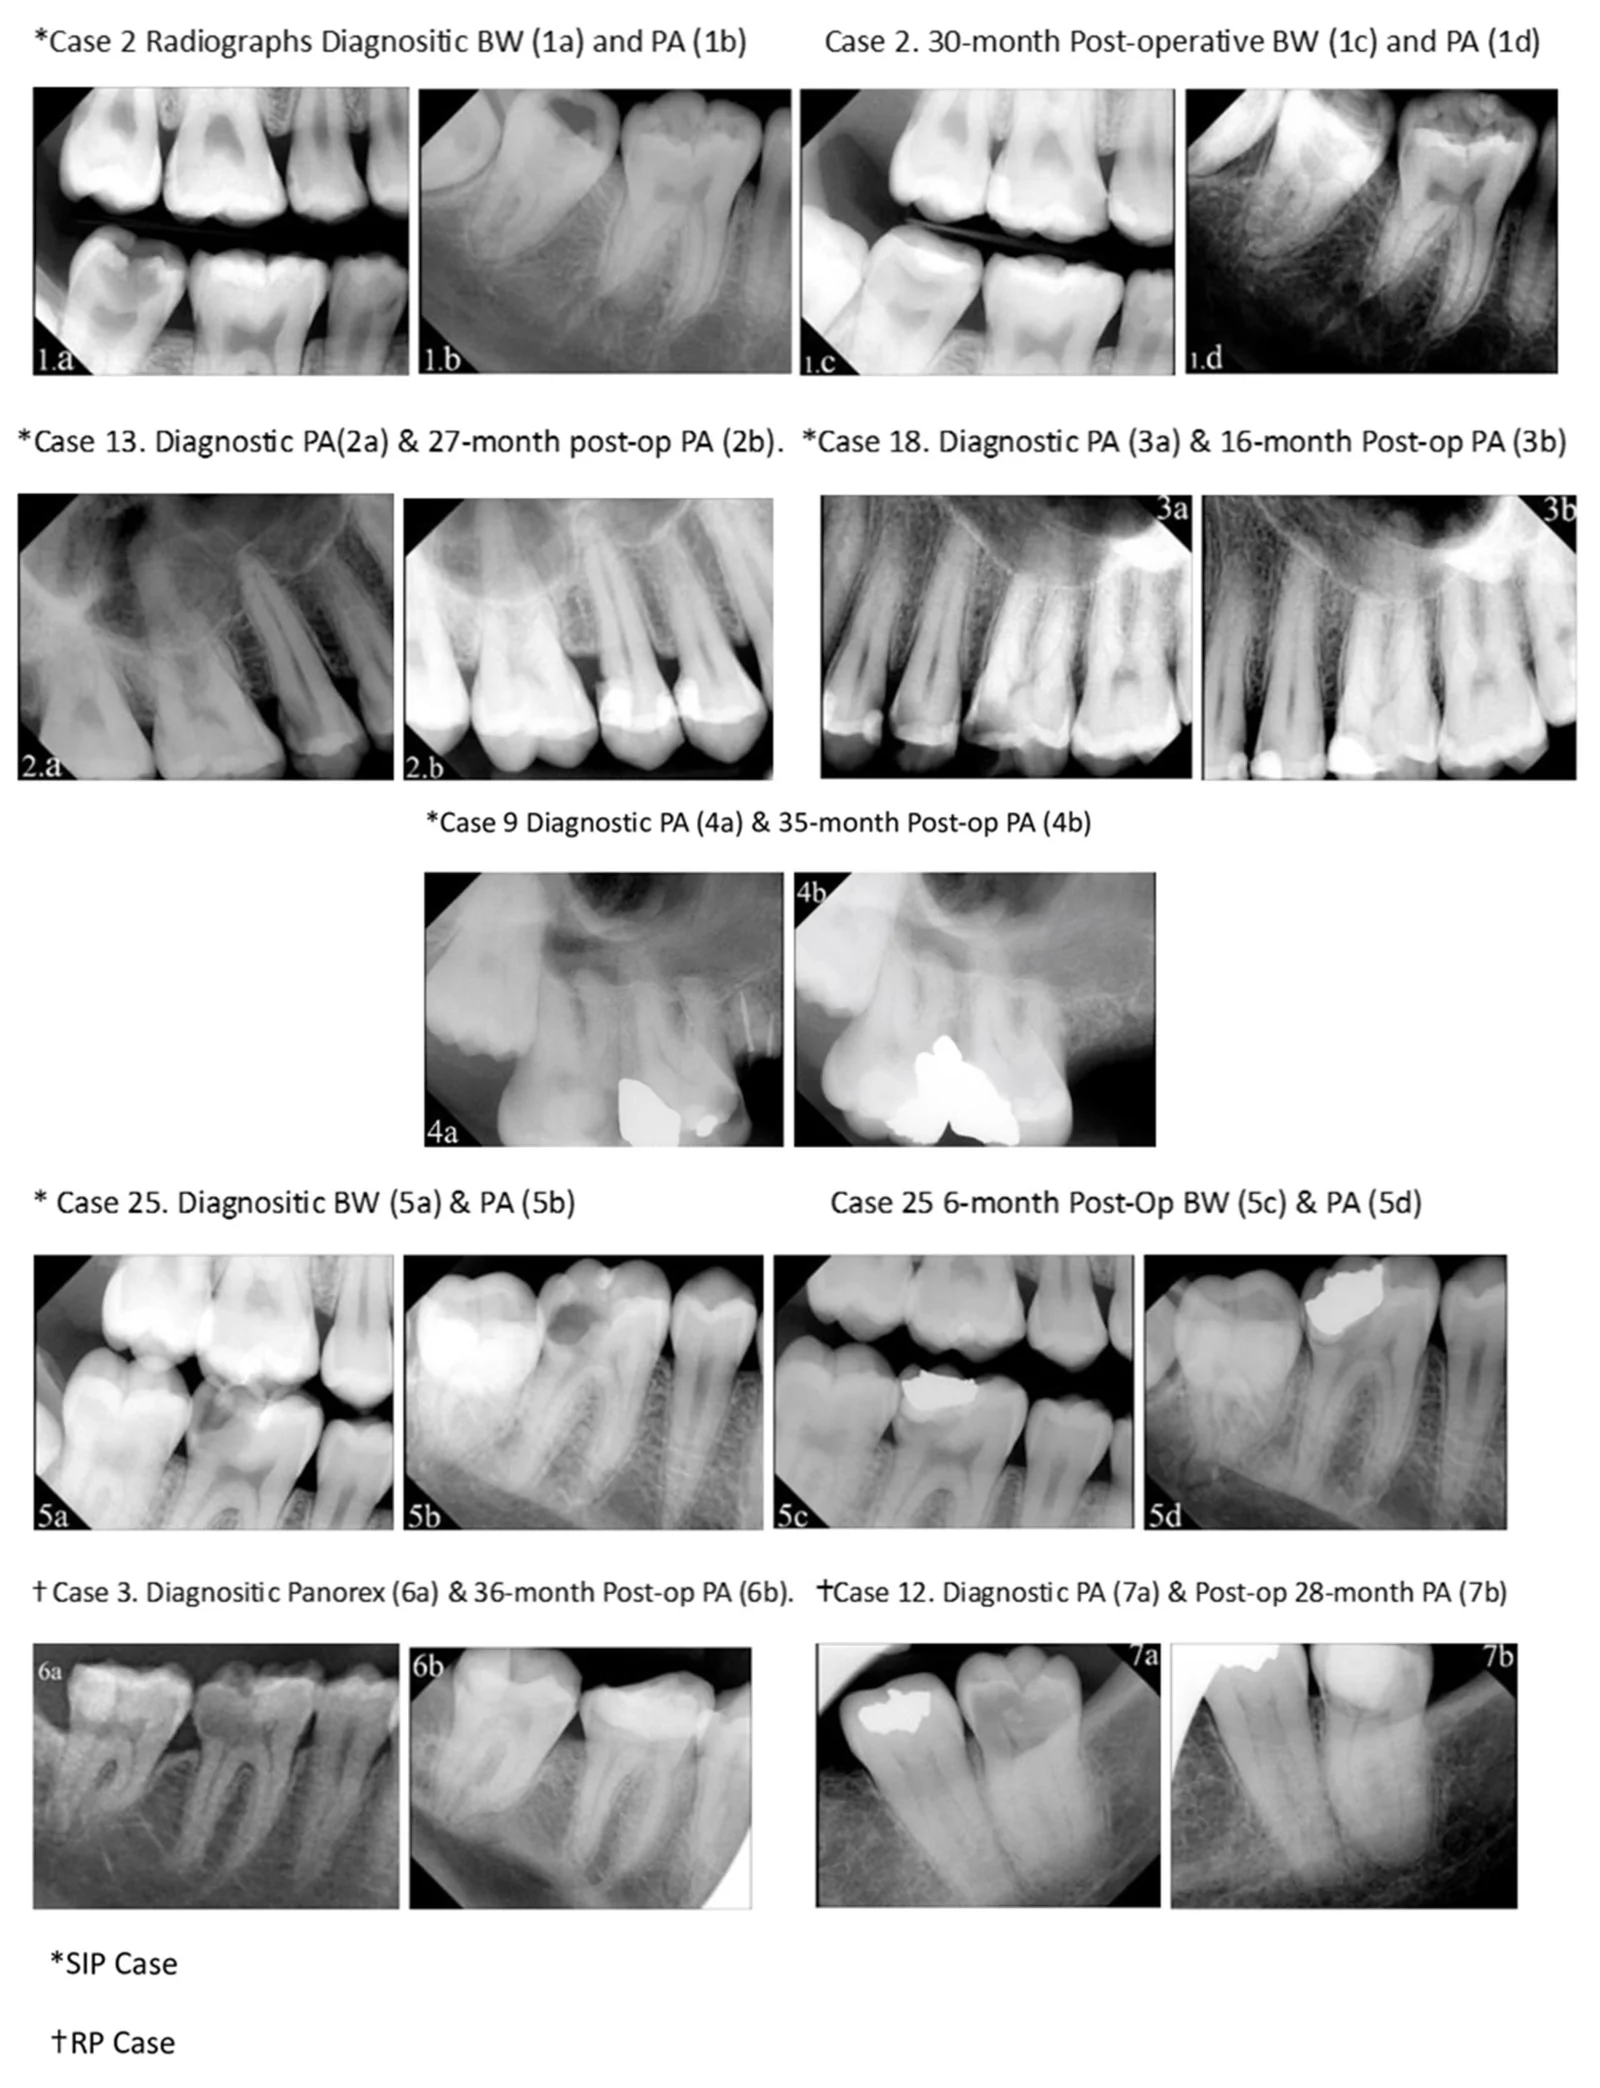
Selected case radiographs.
